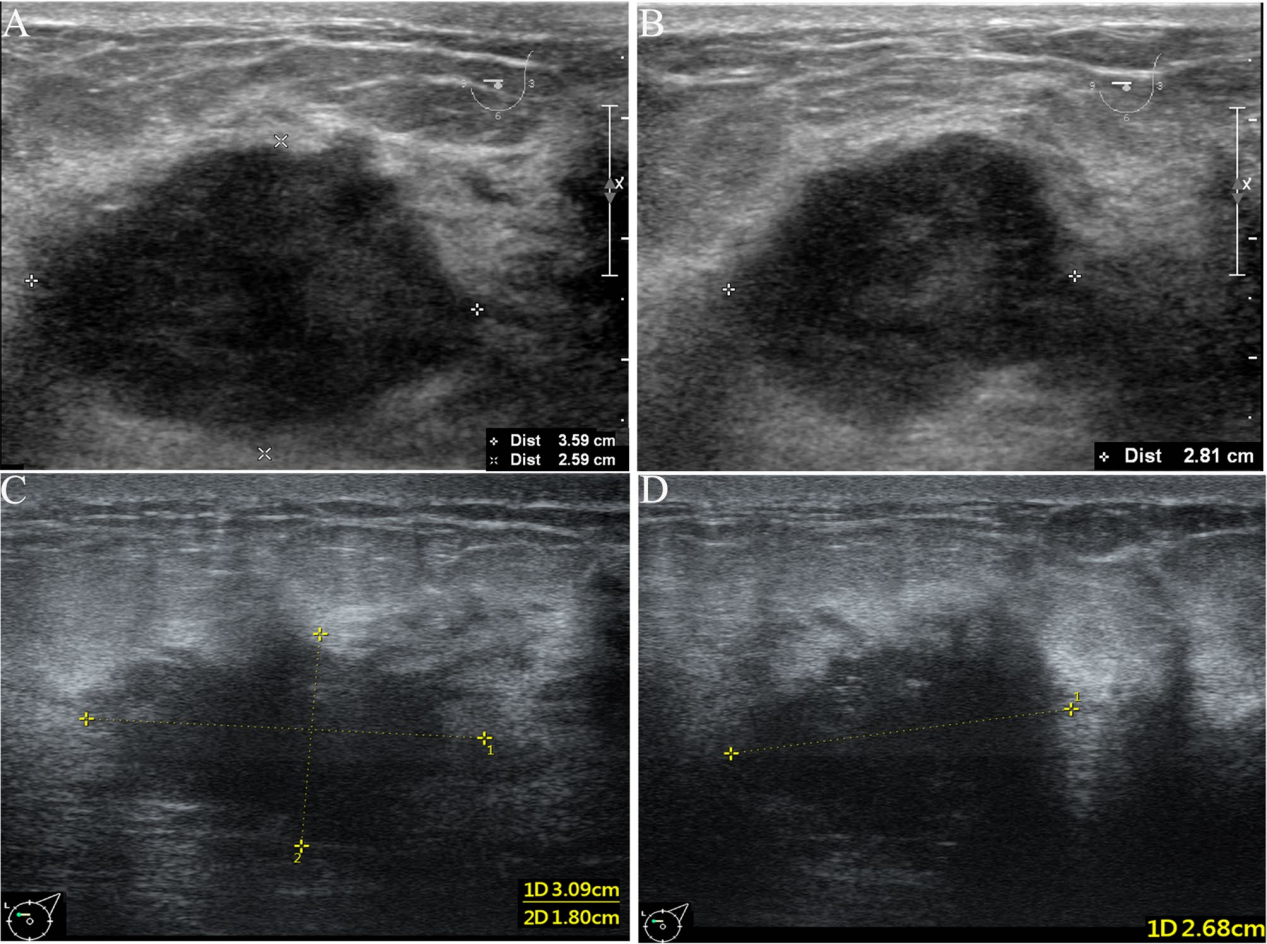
Typical examples of using this nomogram to predict pCR. Images of a female patient (≥ 45 years old, 0 point) with TNBC. (A-B) Baseline grayscale US images of the primary tumor showed a hypoechoic mass measuring 36*26*28 mm (T2 stage, 18 points) with the unchanged posterior acoustic pattern (0 point). (C-D) Grayscale US images of the tumor after two NAC cycles showed the tumor measuring 31*18*27 mm with a 43% calculated reduction in tumor volume (0 point). The total points were 18 (0 + 18 + 0 + 0), the corresponding probability of pCR was less than 10%. This patient completed the neoadjuvant systemic therapy, and followed by radical mastectomy, with a surgical pathology evaluated as non-pCR. US, ultrasound; NAC, neoadjuvant chemotherapy; TNBC, triple negative breast cancer; pCR, pathologic complete response
